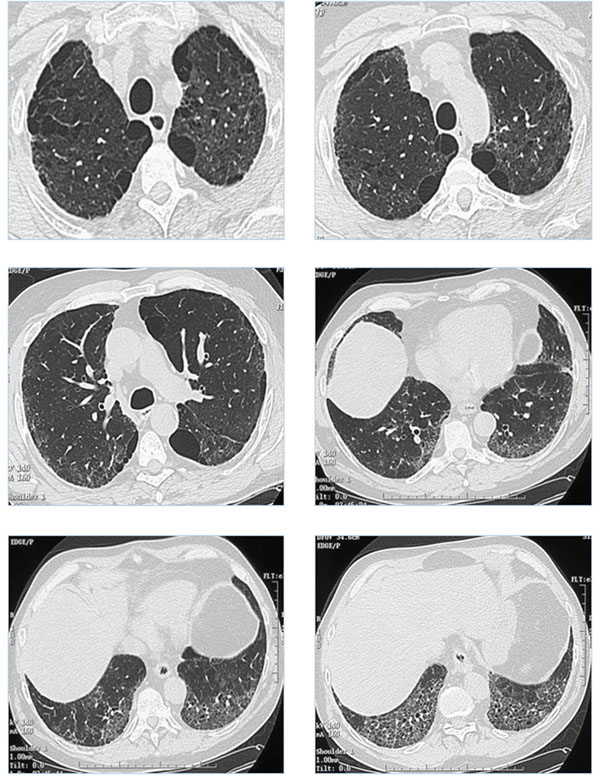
High resolution computed tomography (HRCT) of the chest demonstrating predominantly paraseptal emphysema of the upper areas of the lungs, and interstitial lung disease of the basal regions, with intralobular reticulation and ground glass opacities.
